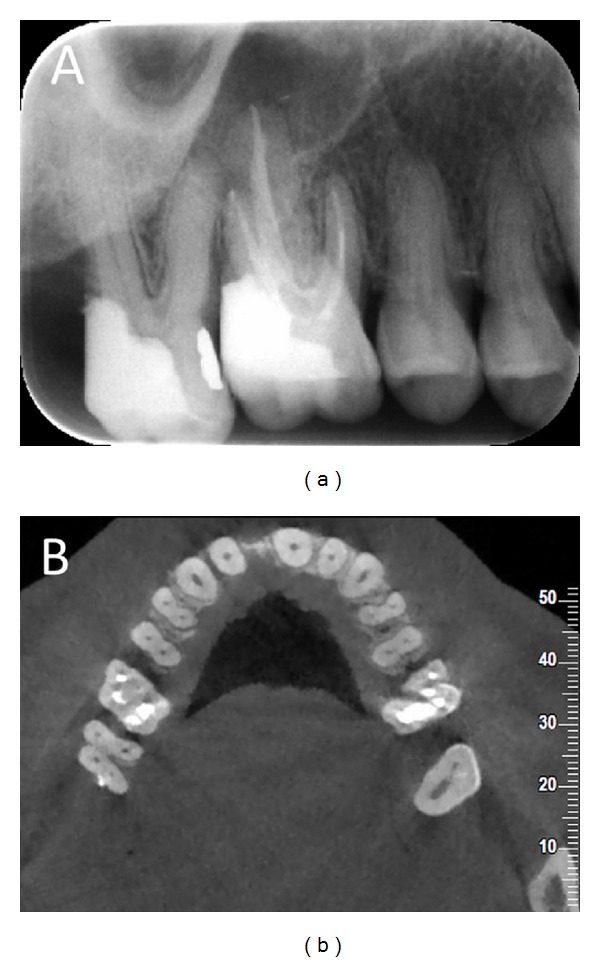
(a) Preoperative radiography showed that the tooth had two roots and no periapical radiolucency. (b) CBCT image revealed the absence of palatal root and two roots with two canals each.
